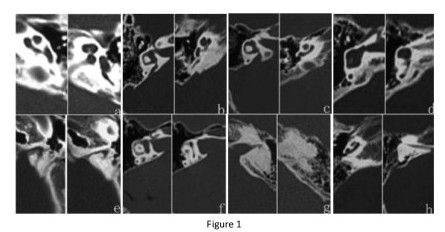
Typical CT scan images of MD, EVA with MD, EVA, and other inner ear malformations. a. MD; b. EVA with MD; c. EVA; d. common cavity (a cystic cavity representing the cochlea and vestibule without showing any differentiation into cochlea and vestibule); e. narrow internal auditory canal; f. internal auditory canal enlarged; g. inner ear ossification; h. cochlear, vestibular, and semicircular canal hypoplasia.
